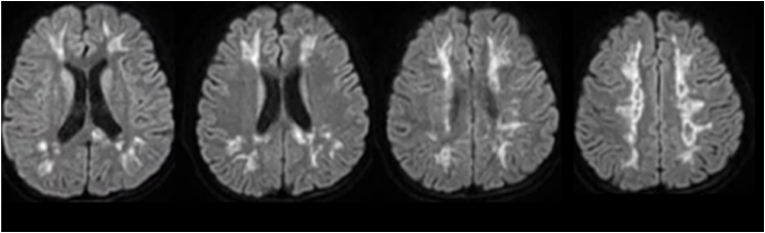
MRI Brain T2-FLAIR (axial) showing white matter hyperintensities in the centrum semiovale, corona radiate and splenium of corpus callosum.
